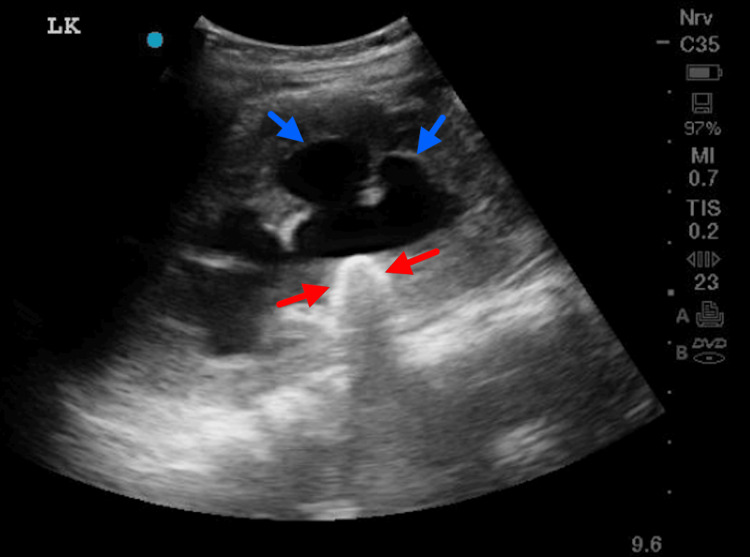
Ultrasound of the left kidney demonstrating renal calculi with hydronephrosis The blue arrows point to the dilated renal calyxes, and the red arrows point to the renal calculi with distal hypechoic shadows.
